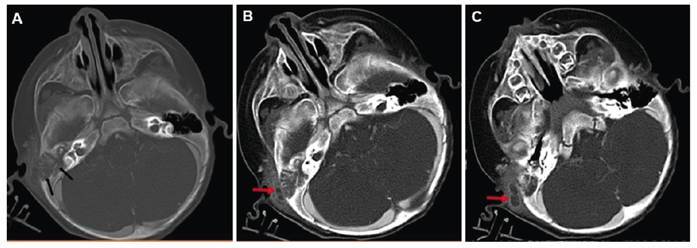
Axial section contrast-enhanced temporal computed tomography images show mastoid cell destruction and diffuse density enhancement (arrows) in the bone window (A) and peripherally contrasted abscess localization extending from the preauricular area to the sternocleidomastoid muscle (arrows) in the soft tissue window (B and C).
